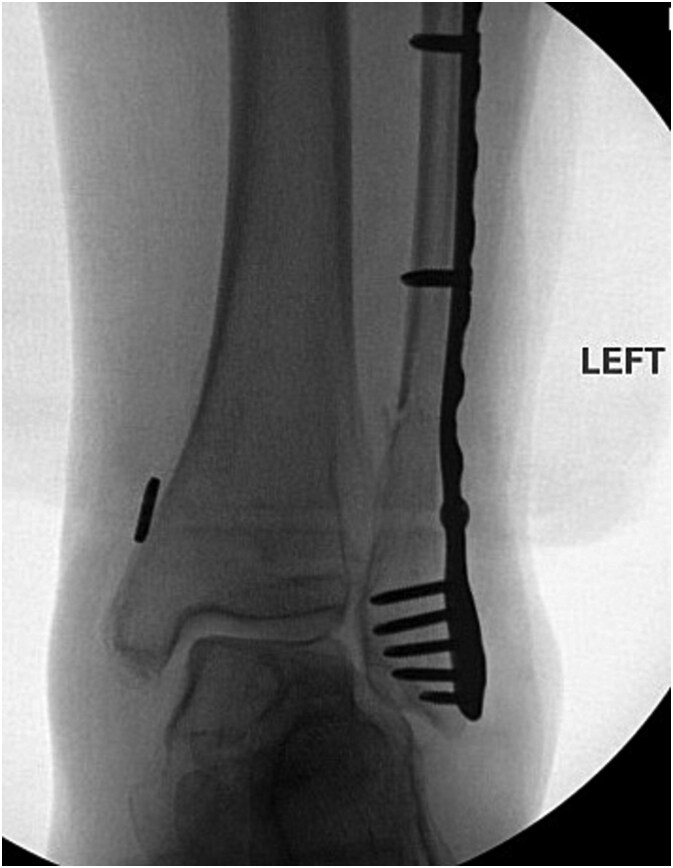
Anterior–posterior post-operative X-ray demonstrating fixation of left Weber C fibular fracture.
